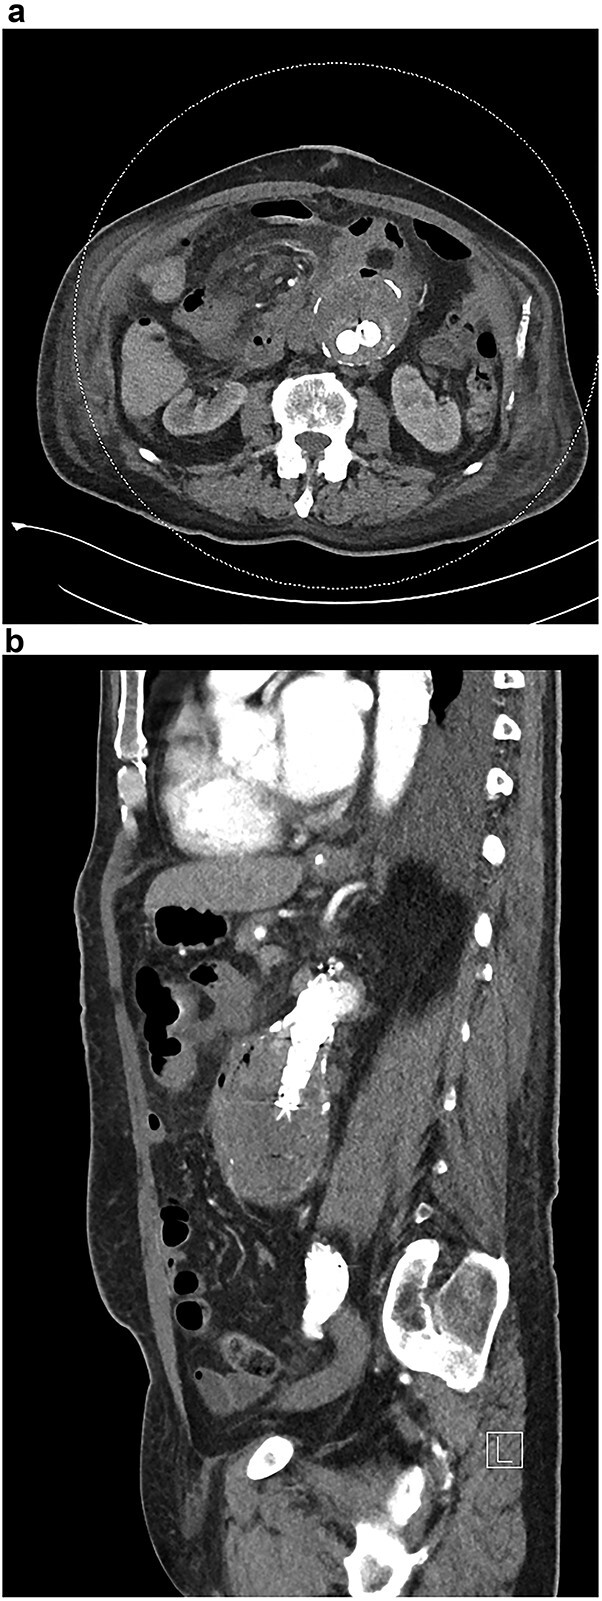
(a and b) CT angiogram after EVAR demonstrating endoleak.
